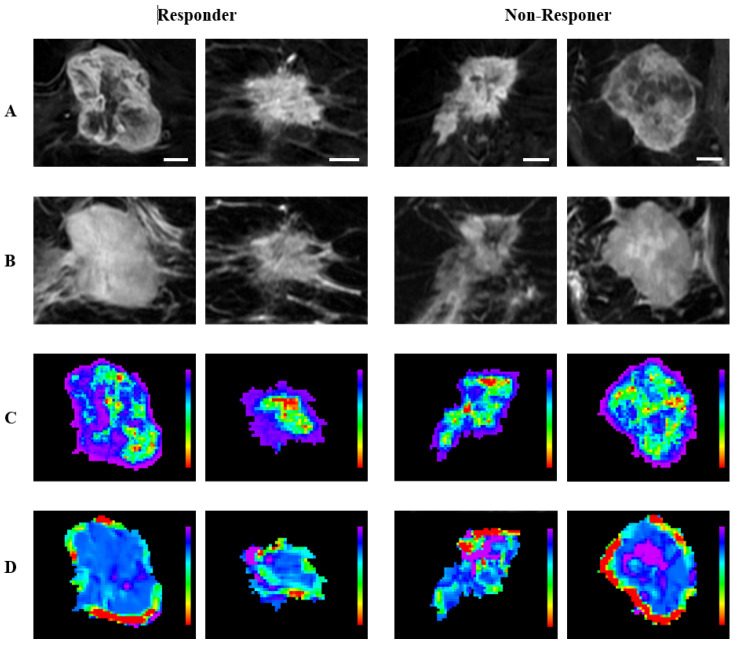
Representative pre-treatment MR images and parametric maps for criterion 2. Each column represents a different tumor corresponding to responder or non-responder. (A) CE-T1 images; (B) T2 images; (C) parametric maps of (CE-T1 × Intra) GLCM_MCC; (D) parametric maps of (T2 × Intra) GLCM_ClusterShade. The CE-T1 images include a 10 mm scale bar. Parametric maps are color-coded, with (C) ranging from 0.8 (red) to 1 (purple) and (D) ranging from −9000 (red) to 3000 (purple).
